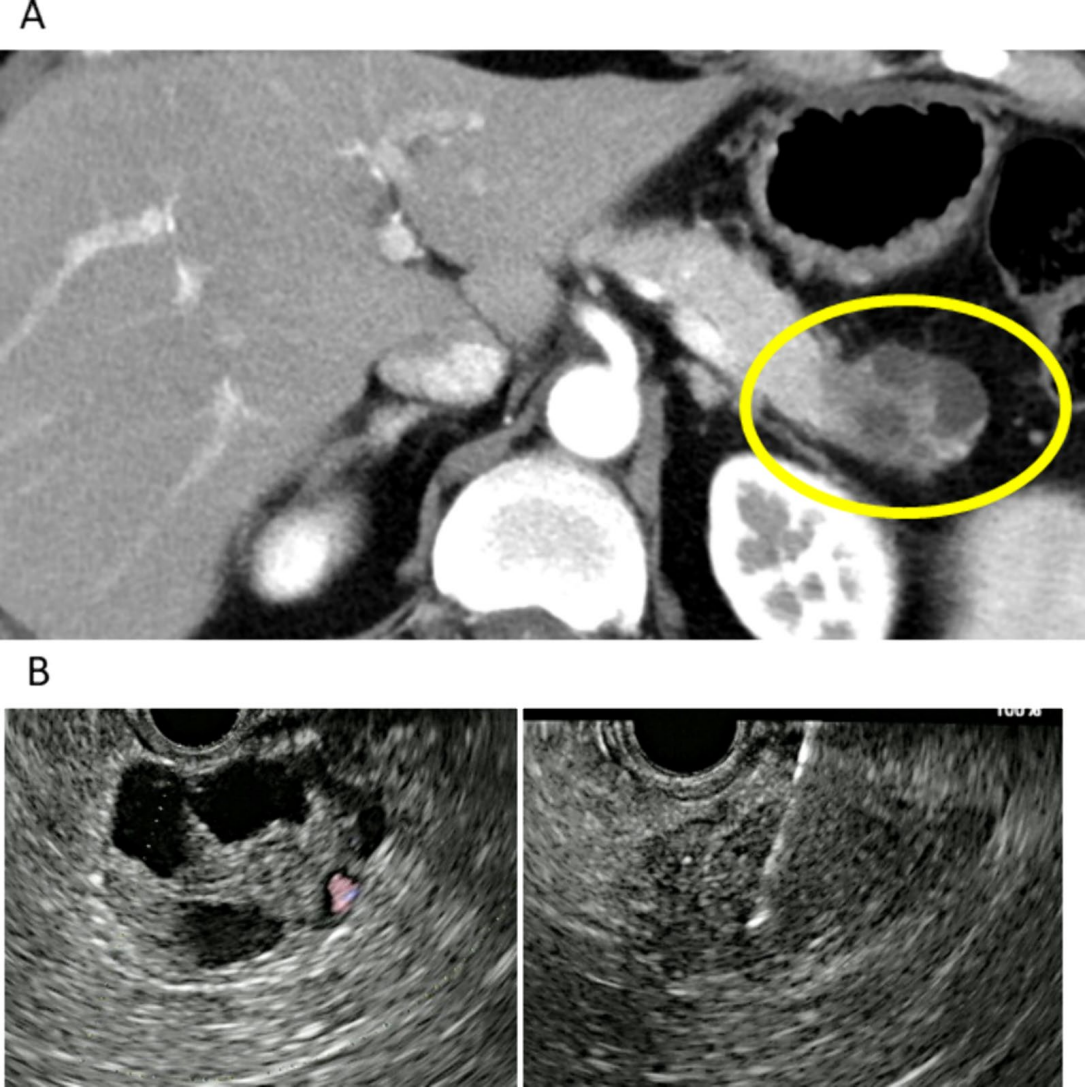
A Enhanced computed tomography and B endoscopic ultrasonography at the initial diagnosis. A The yellow circle shows the primary tumor. B The left panel shows a cystic lesion, and the right panel shows endoscopic ultrasonography-guided fine needle aspiration for a solid tumor
